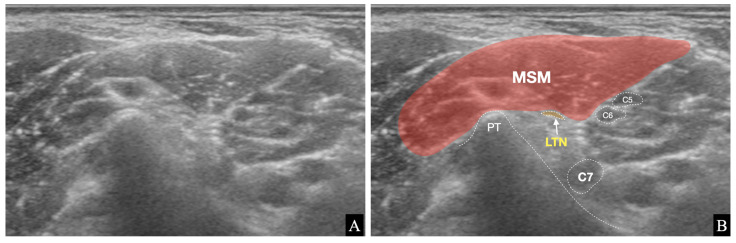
A case that the long thoracic nerve (LTN)’s location is outside of the middle scalene muscle (MSM). (A) A pure ultrasound image without indications of structures. (B) The posterior tubercle (PT), MSM, LTN, and C5–7 nerve roots are indicated on the ultrasound image.
